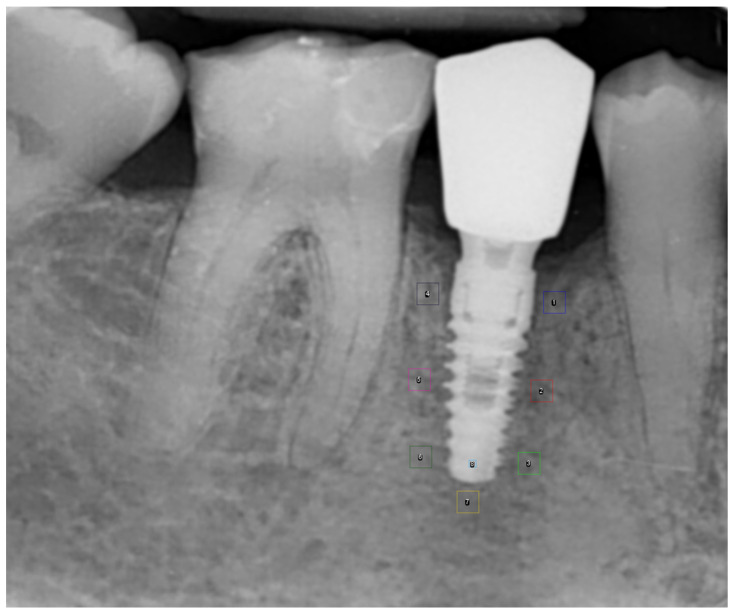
Periapical radiograph showing the position of AOIs from AOI_1 to AOI_8.
